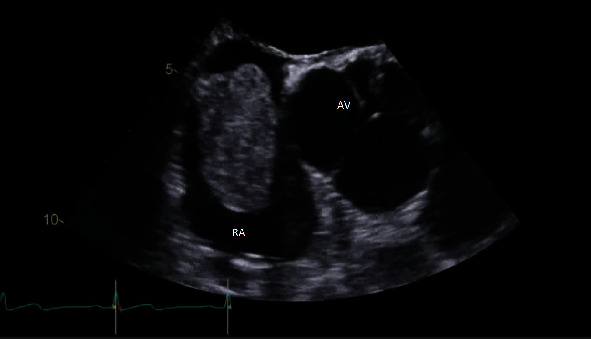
Transoesophageal echocardiogram mid oesophageal aortic valve short axis view demonstrating an echogenic mass in the right atrium (RA) and aortic valve (AV).
